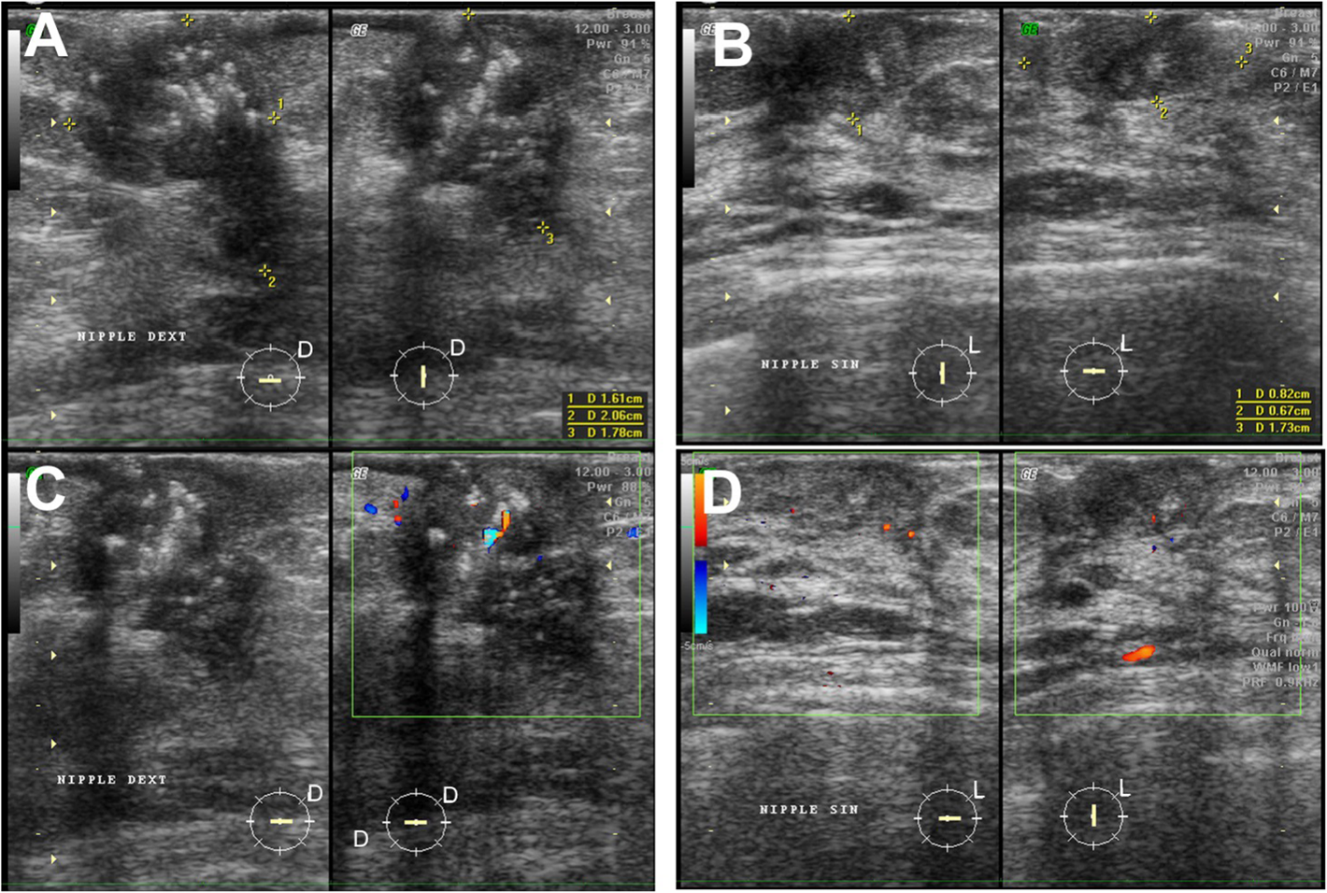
Ultrasound images showing hypoechoic lesion with calcification measuring around 1.61 × 2.06 × 1.78 cm at right retro-papilla (a) and an amorphic, irregular lesion measuring 0.82 × 0.67 × 1.73 cm located at the left retro-papilla (b) with relative hypervascularization at the right and left nipple-areolae complexes (c, d, respectively)
